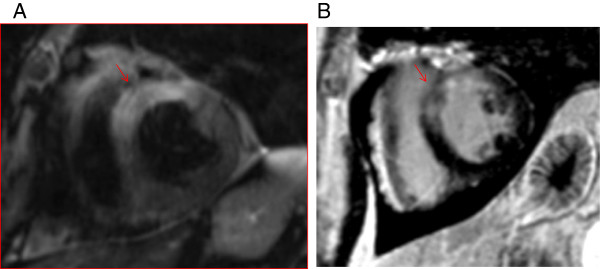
CMR short axis images of the mid left ventricle. T2-weighted image (A) demonstrates bright myocardium (arrow) suggesting edema in the anterior and anteroseptal wall. Late gadolinium enhancement (B) (arrow) corresponds with the territories of bright myocardium on T2-weighted imaging, suggesting edema or a combination of edema and fibrosis.
